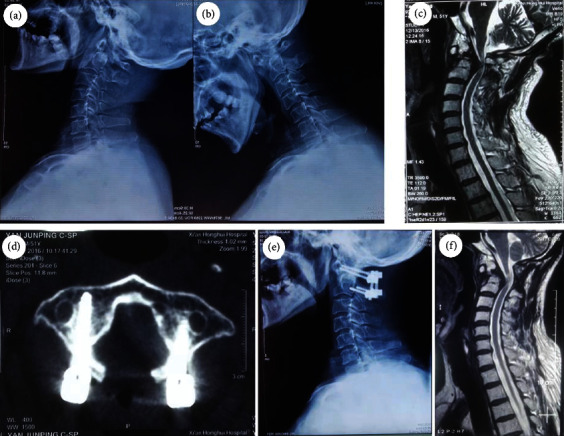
A 61-year-old female with single-level lumbar OVCF treated by unilateral PKP: (a, b) dynamic X-rays show atlantoaxial dislocation and reduction; (c) preoperative dynamic MRI shows evidence of spinal compression after atlantoaxial reduction; (d) postoperative CT shows atlas arch resection; (e) postoperative X-ray shows atlantoaxial reduction; (f) postoperative MRI shows spinal cord decompression.
